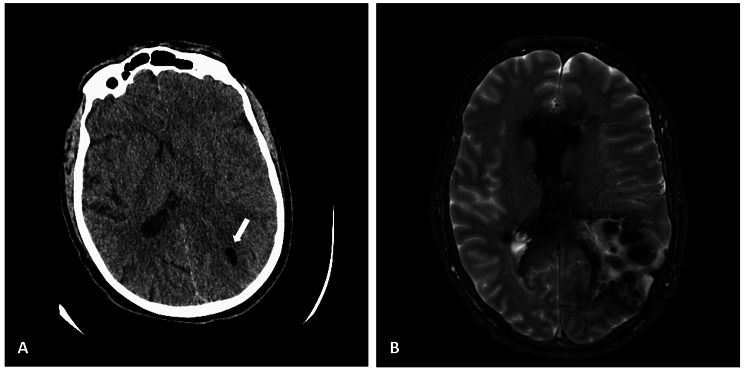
(A) CT scan at presentation demonstrating 1.2 cm air collection in left parietal lobe (arrow). (B) Brain MRI approximately 8 h later with large (7.3 × 6.1 × 5.3 cm) fluid-gas collection extending into the ventricular system
